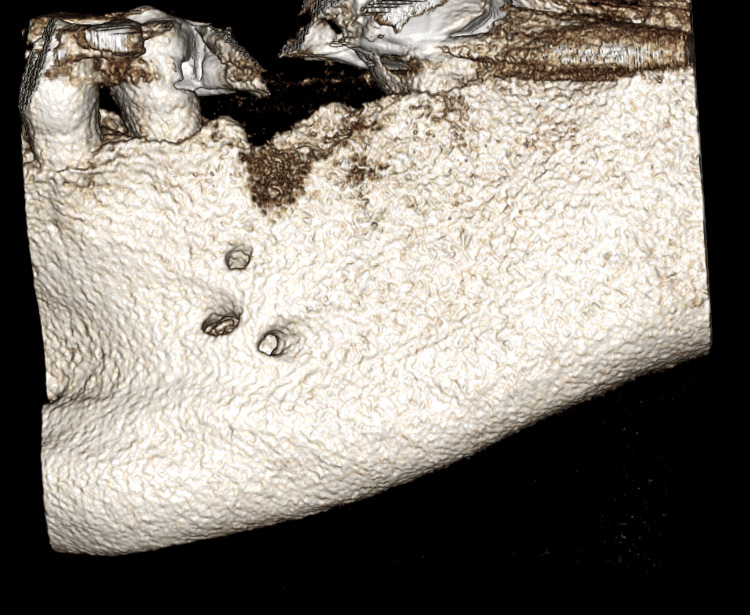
Volumetric reconstruction of CBCT scan showing three mental foramina.
